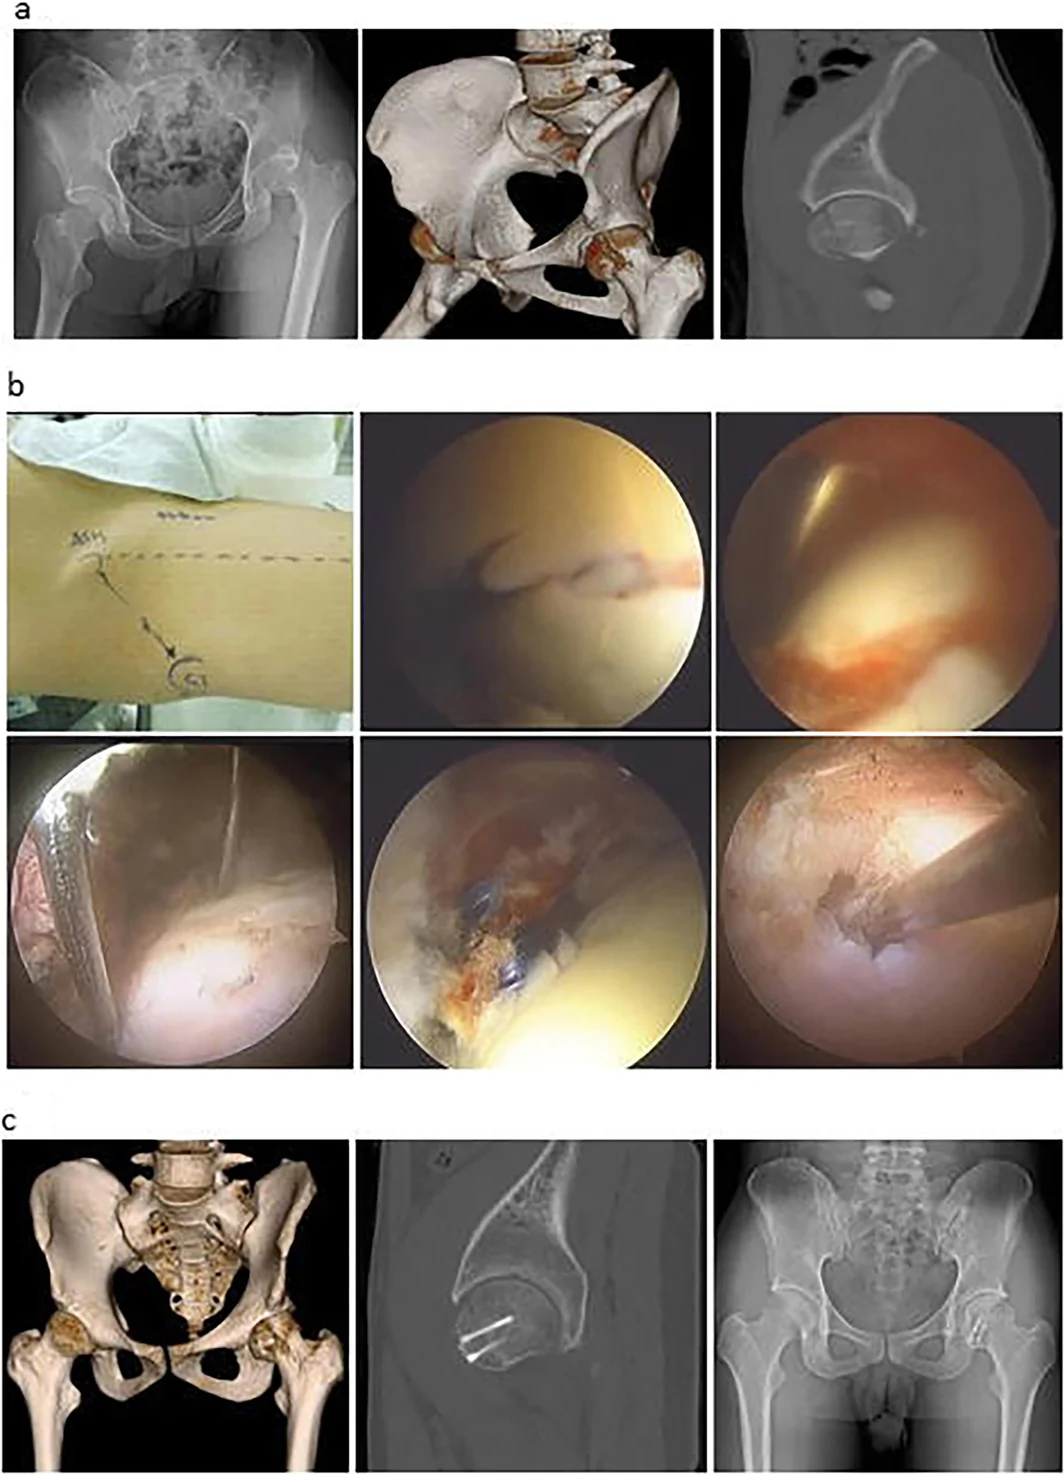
A 21‐year‐old female with a left Pipkin Type I fracture. (a) Preoperative imaging; (b) intraoperative arthroscopy–assisted reduction and fixation technique. Preoperative surface marking and identification of the femoral artery using ultrasonography for safe portal placement. Arthroscopic view from the anterolateral portal showing the displaced femoral head fragment. Use of the “joystick technique” with a probe and elevator to manipulate the fragment. Achievement of fracture reduction under direct visualization. Percutaneous insertion of guide wires and definitive fixation with headless compression screws. Final arthroscopic inspection confirming that screws are properly countersunk beneath the subchondral bone with no intra‐articular protrusion; (c) 6‐year follow‐up showing fracture healing and joint congruency without screw migration.
